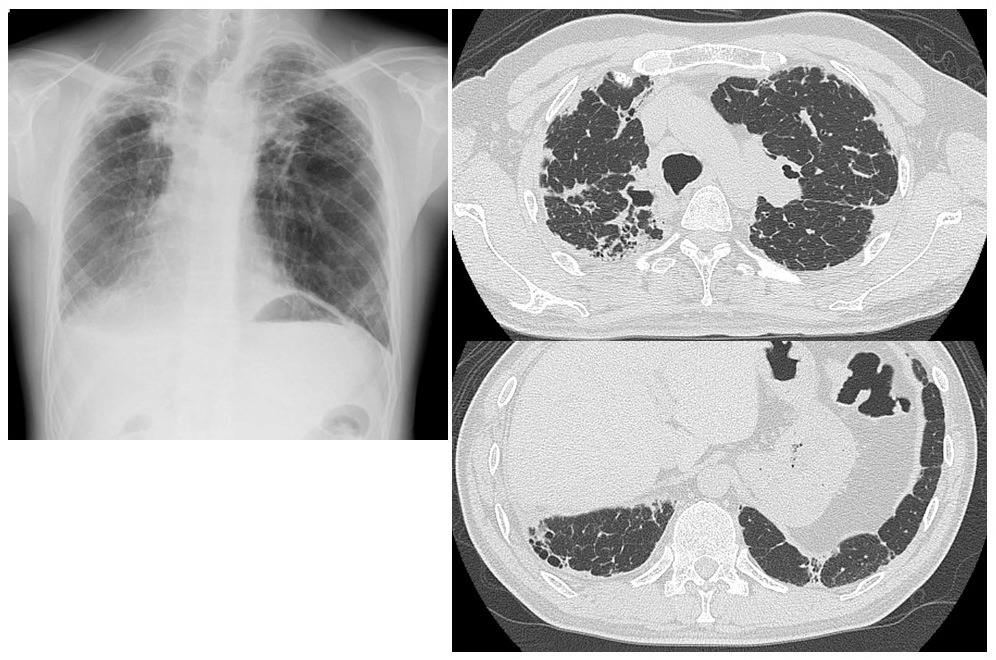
Chest radiograph and CT scans of Case 1 showing elevation of bilateral hilar opacities and subpleural reticular opacities in the bilateral lung fields.
